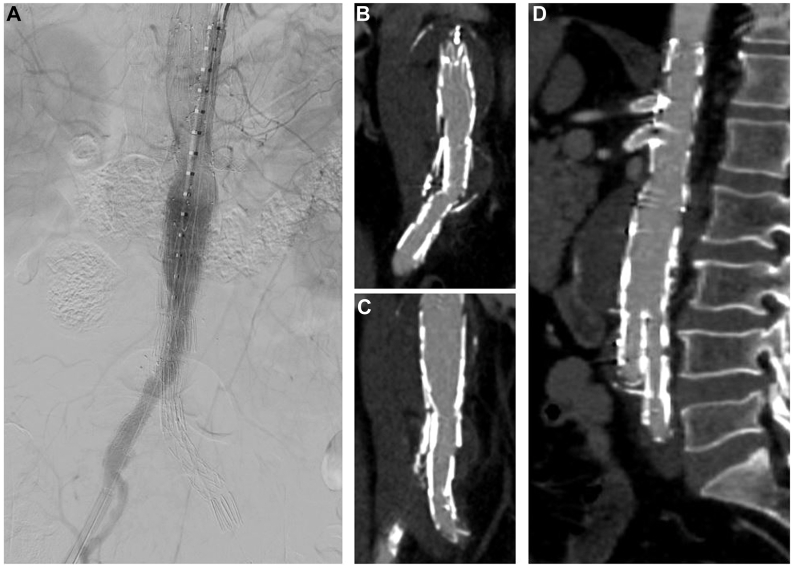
Completion angiography demonstrates antegrade right lower extremity flow via the right iliac limb deployed through the *in situ* laser fenestration (ISLF) (A). On postoperative computed tomography angiography (CTA), both the right iliac limb (B) and the left iliac limb (C) had inline flow with successful exclusion of the aneurysm sac (D).
